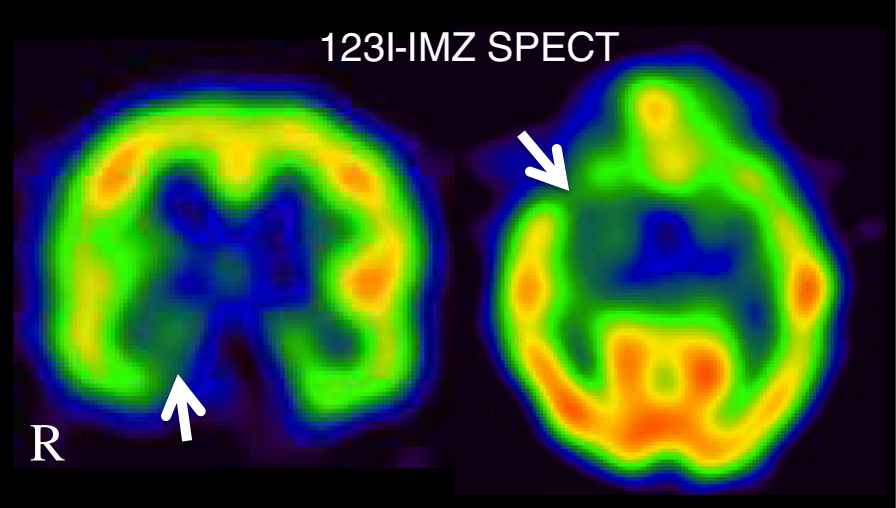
SPECT with ^123^I-iomazenil 10 days after admission revealed decreased benzodiazepine receptor binding in the right medial temporal area (*arrows*)
